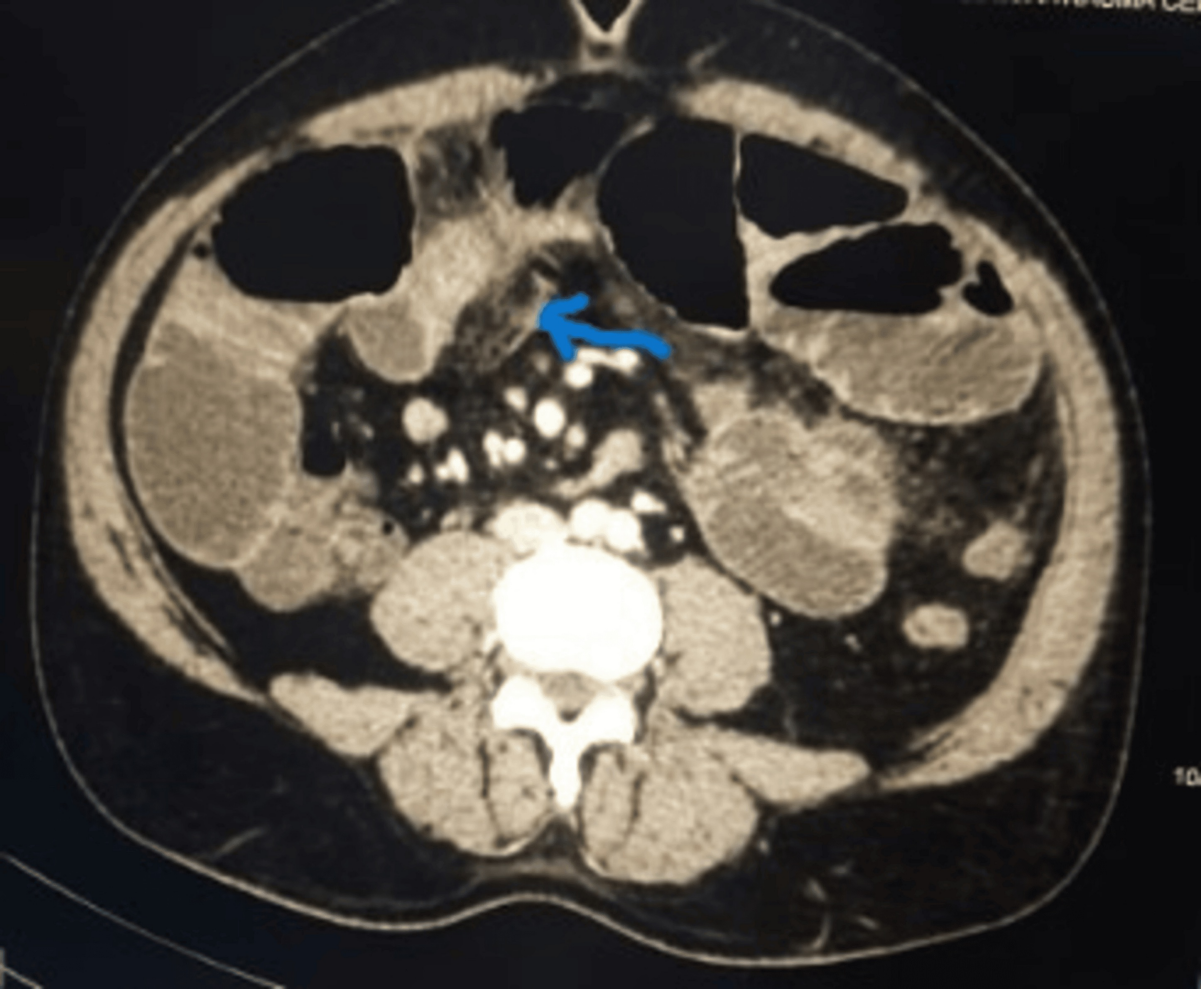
CECT of the abdomen, revealing an inflammatory mass (blue arrow) attached to the anterior abdominal wall, accompanied by adjacent fat stranding and distended small bowel loops that abruptly collapse at the distal jejunum, with minimal peritoneal fluid present CECT: contrast-enhanced computed tomography
